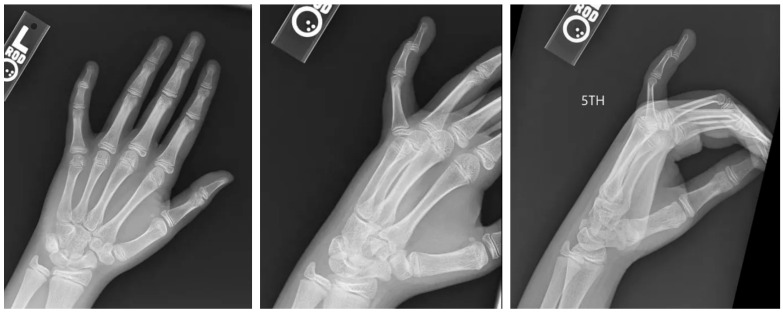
Initial radiographs. AP, oblique, and lateral views of the left hand in a skeletally immature individual demonstrating a dislocated distal phalanx of the small finger.
